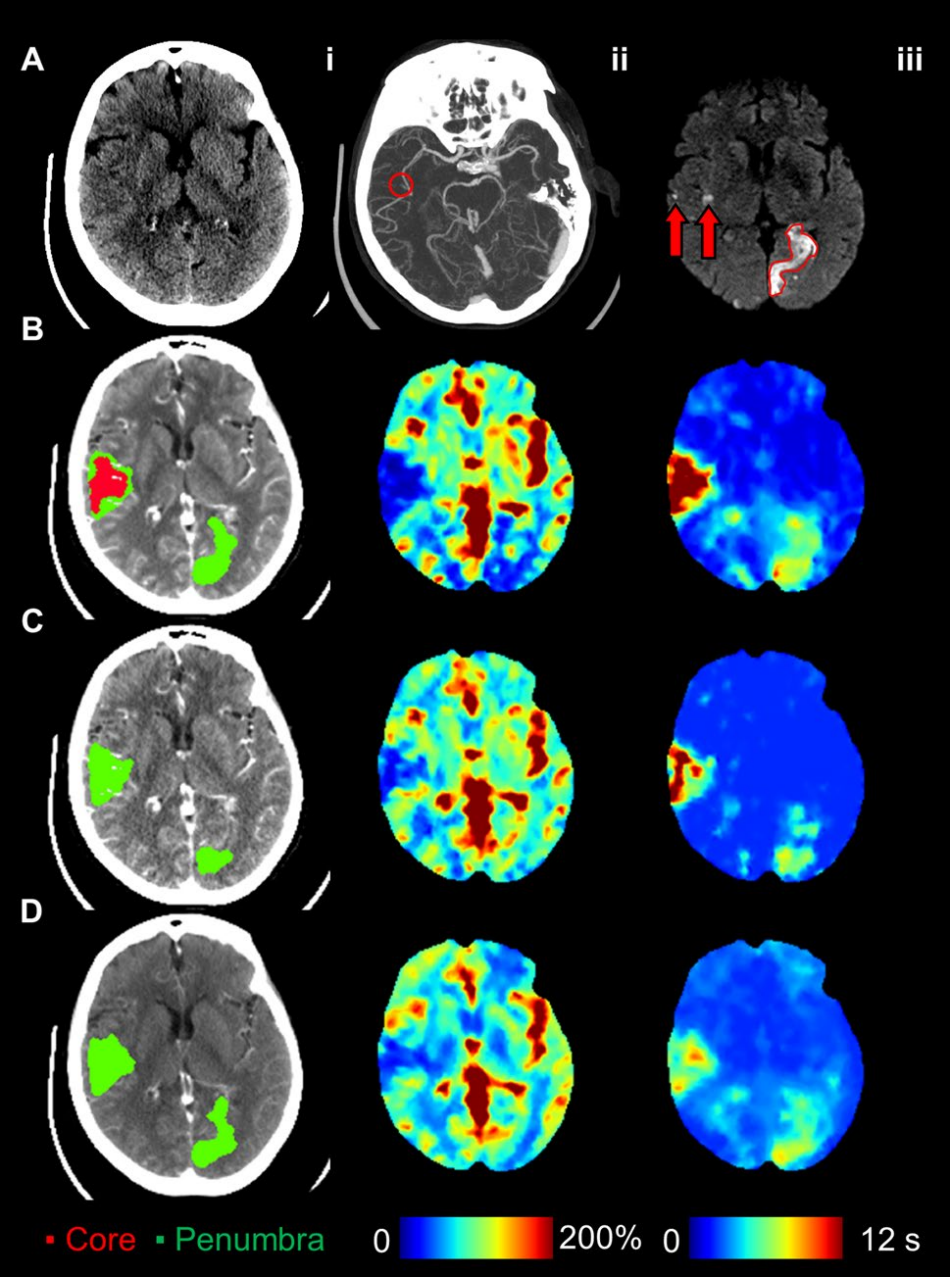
Patient presenting with unknown time from stroke symptom onset, a bilateral occlusion of the right M2 middle cerebral artery (MCA) and left distal posterior cerebral artery (PCA), and an NIHSS of 10. This patient received endovascular thrombectomy for the right M2 occlusion within 53 min of admission CT (mTICI = 3), but the posterior occlusion could not be treated. (A.i) Admission non-contrast CT; (A.ii) maximum intensity projections of CT angiography with the red circle indicating the occlusion site; (A.iii) follow-up diffusion-weighted imaging showing regions of infarction in the right M2 territory (red arrows) and the left posterior brain (red outline). (B.i) CT perfusion ischemic core (red, 19.4 ml) and penumbra (green, 45.5 ml; mismatch ratio of 2.3) derived from cerebral blood flow (B.ii) and Tmax maps (B.iii) indicated a favourable DEFUSE-3 mismatch profile. (C) Similarly, down-sampled CT perfusion (dCTP) indicated a favourable mismatch profile with an ischemic core and penumbral volumes of 4.8 ml and 26.9 ml, respectively (mismatch ratio 5.6). (D) Multiphase CT angiography-perfusion (mCTA-P) agreed with the favourable CTP mismatch profile with ischemic core and penumbral volumes of 1.2 ml and 51.2 ml, respectively (mismatch ratio 44.3). Of note, the indicated lesion volumes were only of the right MCA territory so that the left PCA lesion volumes did not affect the estimation of mismatch.
